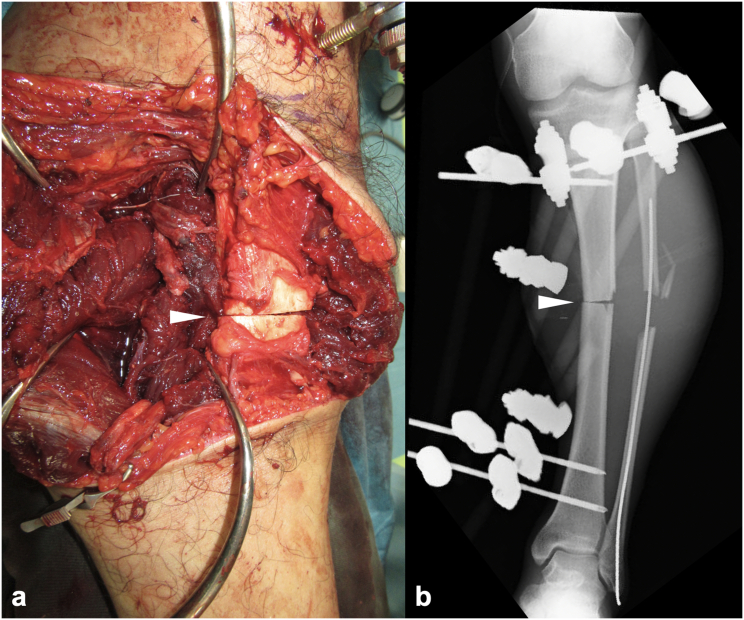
Intraoperative tibial osteotomy and shortening. (a) Intraoperative photograph showing the approx. 4 cm of tibial shortening, stabilized using a modular external fixator (*(white arrowhead*). (b) Post-shortening anteroposterior plain radiograph showing bony contact between fragments (*(white arrowhead*), achieved through external fixation.
